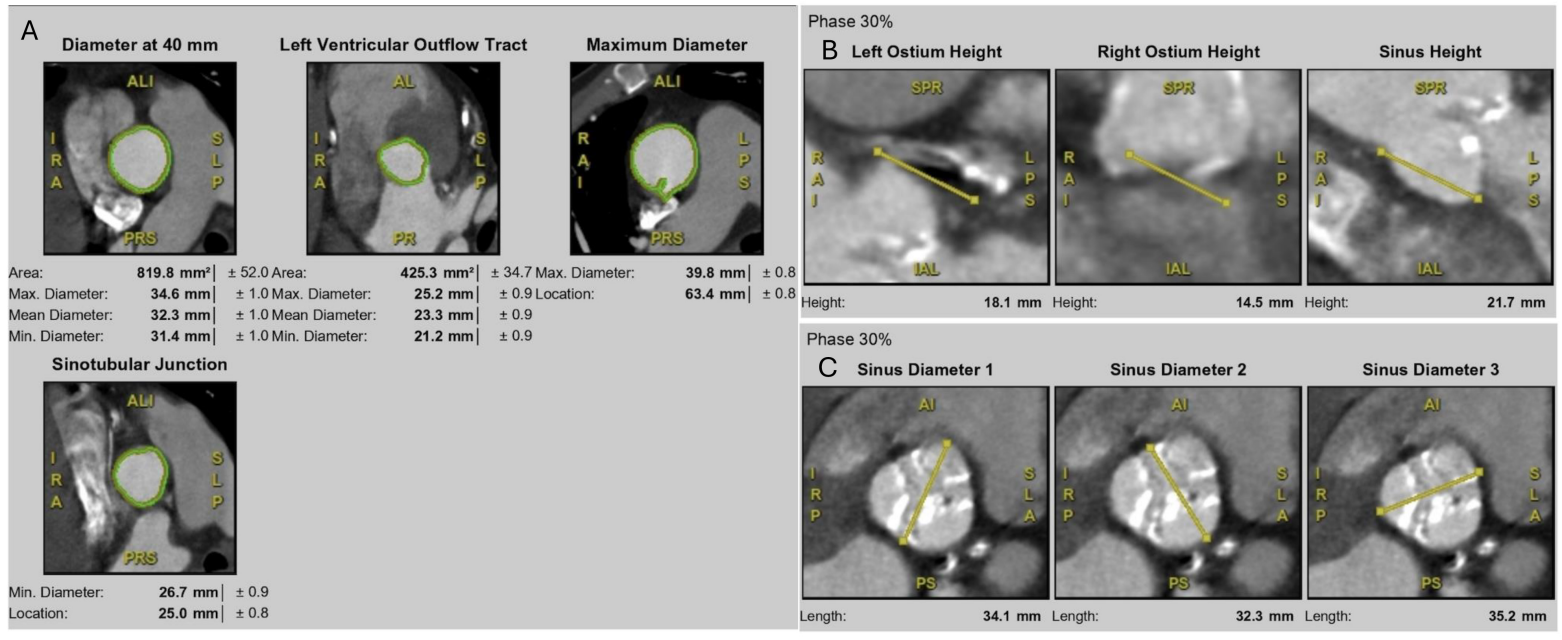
Multidetector CT measurements for TAVI planning. (A) Aortic
